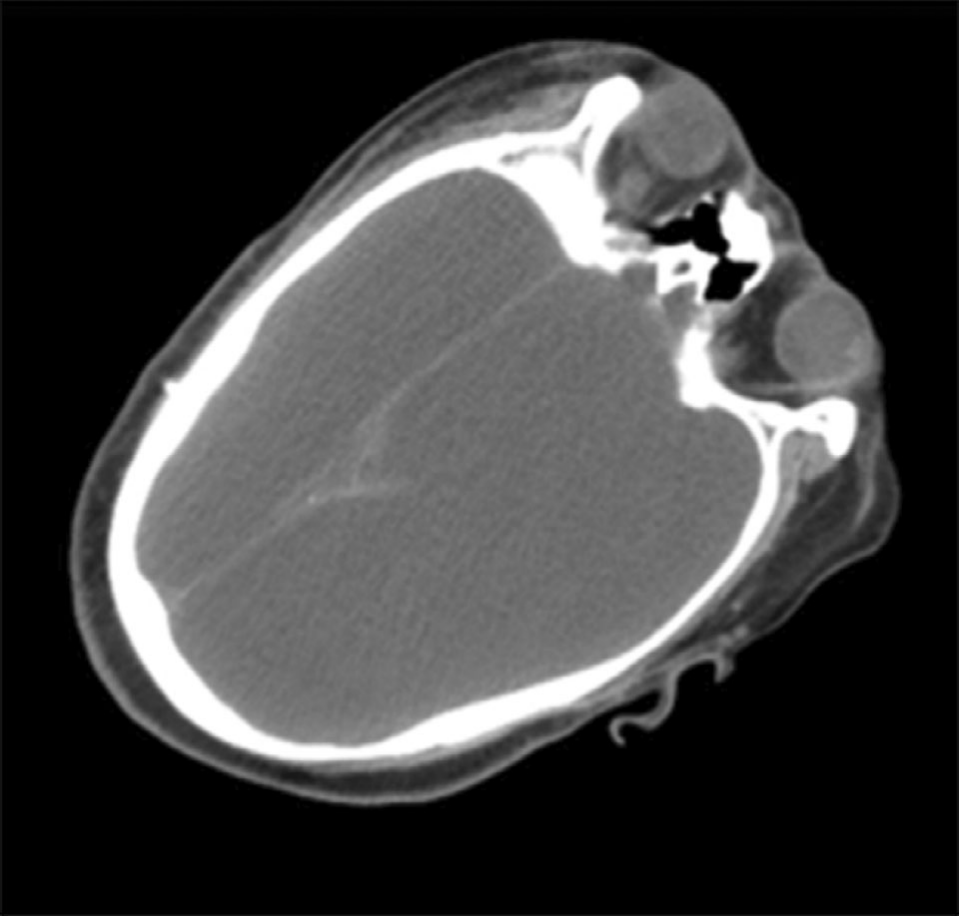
Brain CT scan showing complete replacement of the cerebral hemispheres with fluid.
